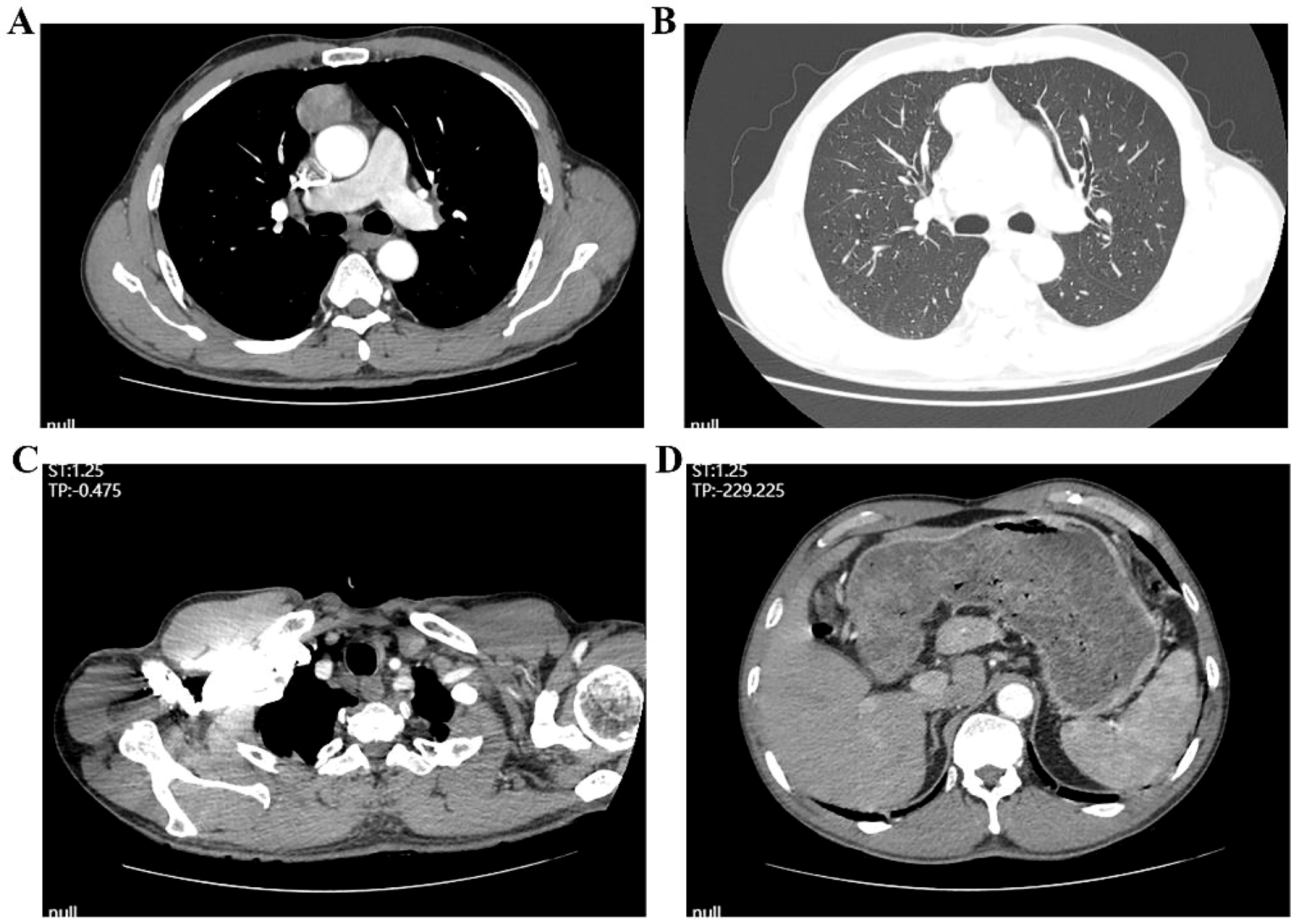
Enhanced chest CT showing esophageal lesions and lymph node metastasis. (A, B) Mediastinal windowing reveals a soft tissue mass within the mediastinum; (C, D) Serial images demonstrate evidence of mediastinal lymphadenopathy.
